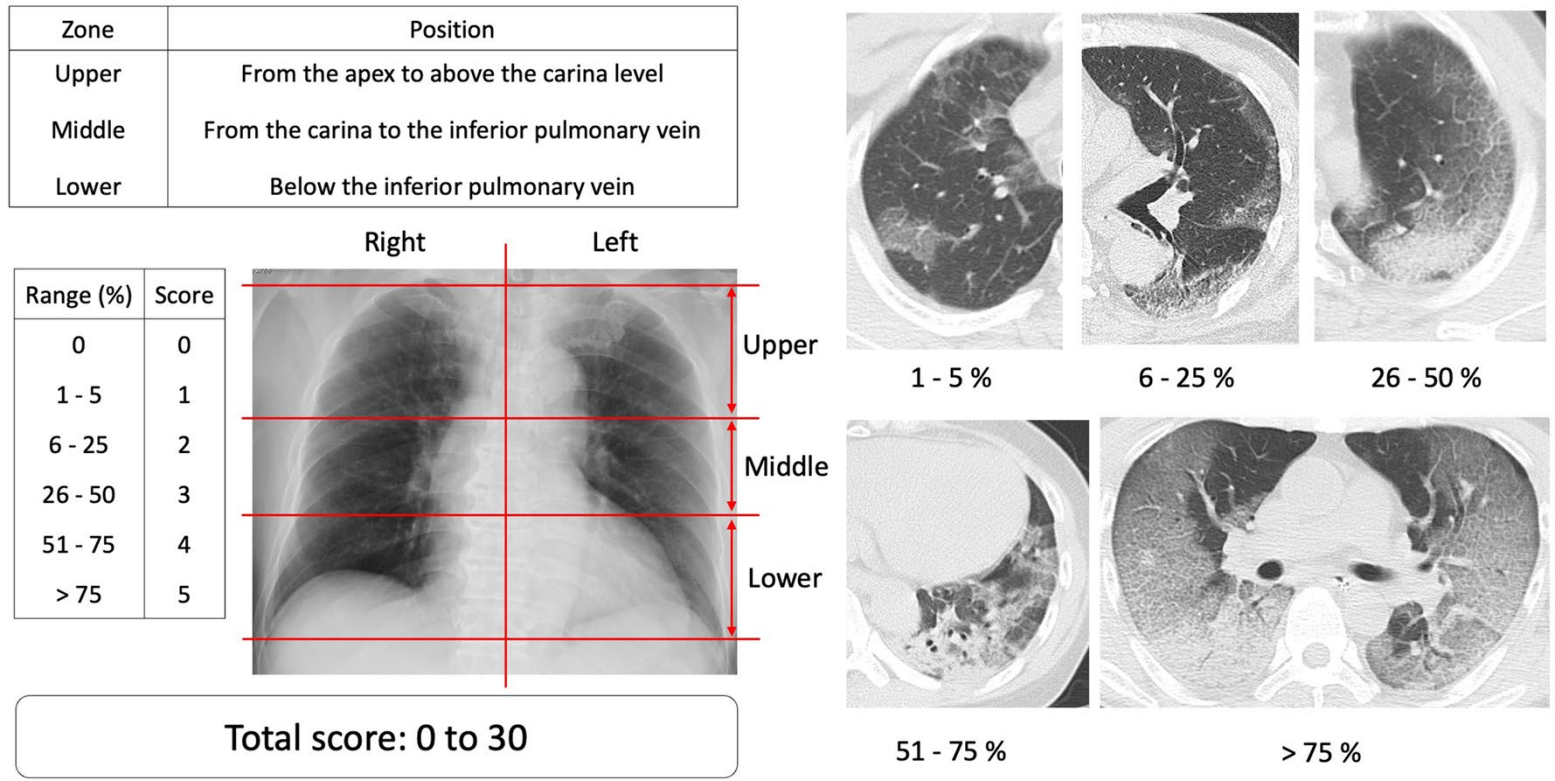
CT scores and axial images by lung zone involvement in COVID-19 pneumonia. The lung area was divided into three zones from the apex to bottom. The upper lung zone extended from the pulmonary apex to the bronchial bifurcation, the middle from the bronchial bifurcation to the right inferior pulmonary vein, and the lower from the right inferior pulmonary vein to the bottom.
